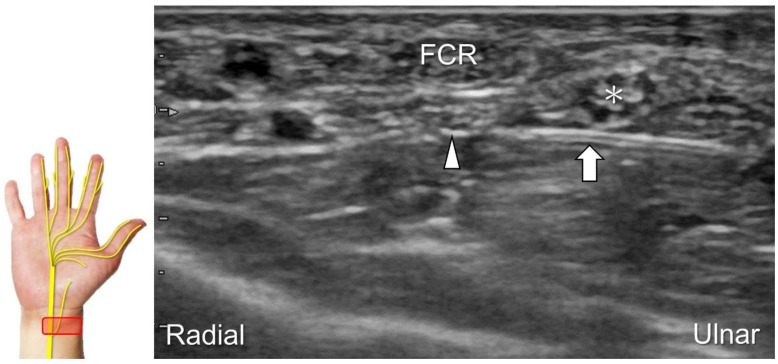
Ultrasound-guided hydrodissection (short-axis view) for the palmar cutaneous branch of the median nerve. Asterisk: median nerve; arrowhead: palmar cutaneous branch of the median nerve; arrow: needle. FCR: flexor carpi radialis tendon.
